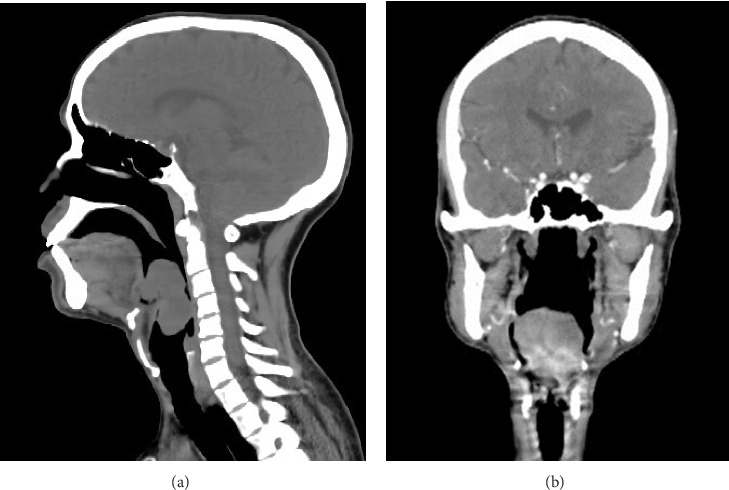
(a) Plain sagittal CT scan showing a well-defined sessile mass originating at laryngeal surface of epiglottis. (b) Coronal postcontrast image shows heterogeneous enhancement.
